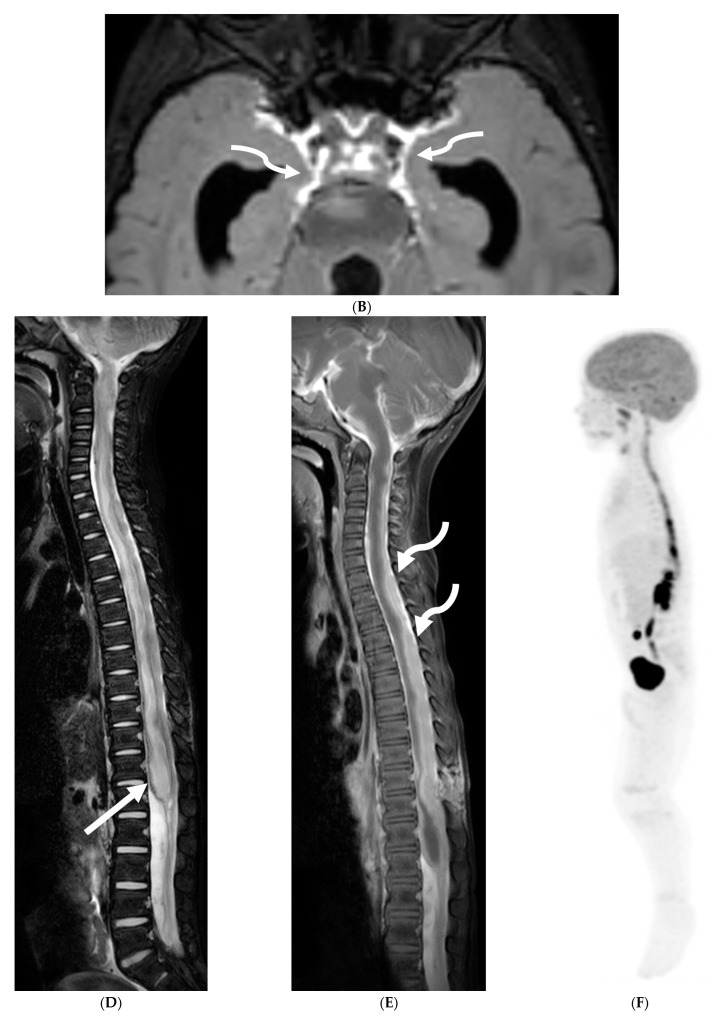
3 weeks follow up: Post contrast sag T1 (A) and axial FLAIR (B), Axial T2 (C), sagittal T2 (D), Post contrast sagittal T1 (E) and PET/CT (F): Leptomeningeal nodular enhancement along posterior fossa, suprasellar and spinal cord (curved arrows) has significantly increased. There is also new/increased signal abnormality in the brain stem and cord (white arrows). Hypermetabolic spine disease is demonstrated on PET/CT. No osseous involvement is identified on the PET scan. Pathology: Diffuse CNS ALK (Anaplastic Lymphoma Kinase)-Positive Histiocytosis. Bone marrow biopsies, US abdomen and skeletal survey negative for extracranial/extraspinal disseminated disease.
